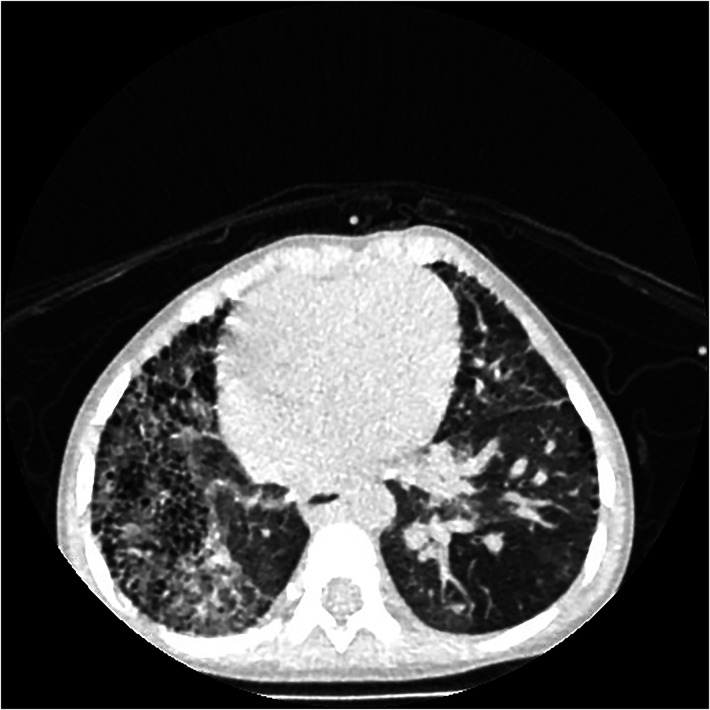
Right proximal interruption of the pulmonary artery with hemoptysis (twice) in a 22-month-old male patient. A transverse view of a plain computed tomography scan shows speckled and patchy shadows in the right lung field, and reticulated shadows, thickened interlobular septa, and small subpleural cystic lucency shadows were observed in both lungs.
